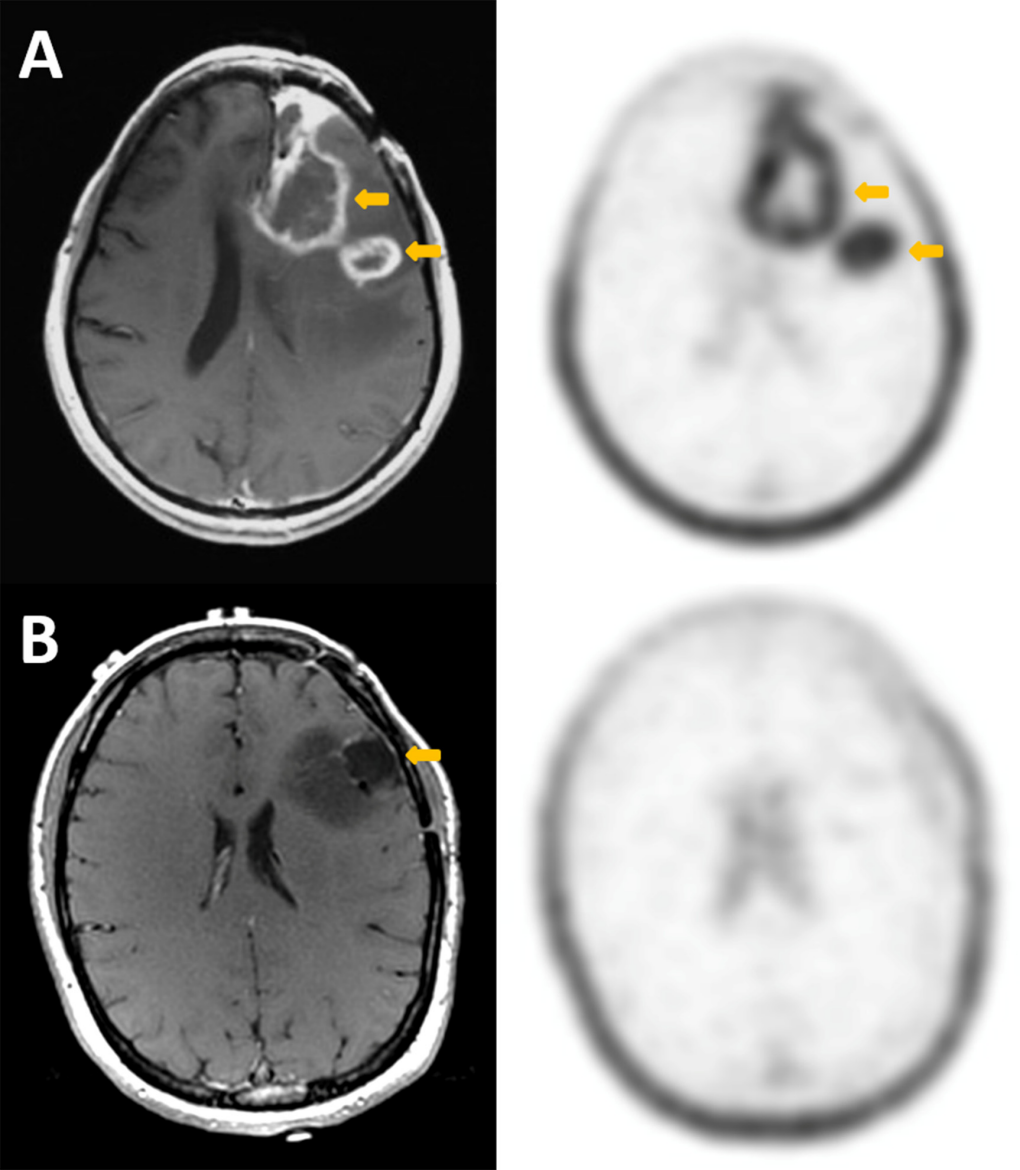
Comparison of axial post-contrast T1 MRI (left column) and 18F-FSPG PET (right column) in the two subjects with primary brain tumor. Subject A has recurrent high-grade glioblastoma, while subject B has an enlarging, partially resected low-grade oligodendroglioma. In each case, the arrows point to the primary lesions. For subject A, there is intense accumulation of 18F-FSPG in the enhancing lesion (SUV 5.2), while in subject B, there is no accumulation of the radiotracer (SUV 0.7) in the non-enhancing lesion. Comparatively, the background brain SUV is 0.1 for both subjects. There is incidental note of prominent physiologic uptake of 18F-FSPG in the scalp.
